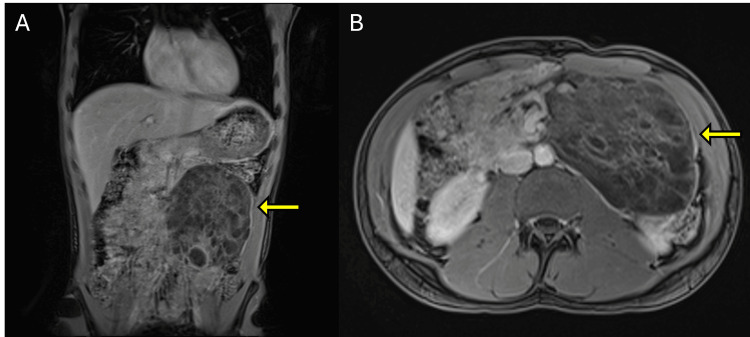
(A) Coronal and (B) axial T1-weighted post-contrast MRI images in the portal venous phase showing a large, multiloculated cystic mass in the left retroperitoneum (yellow arrows). The lesion demonstrates thin, mildly enhancing internal septations without solid enhancing components. These findings are consistent with a macrocystic lymphatic malformation. MRI: magnetic resonance imaging
